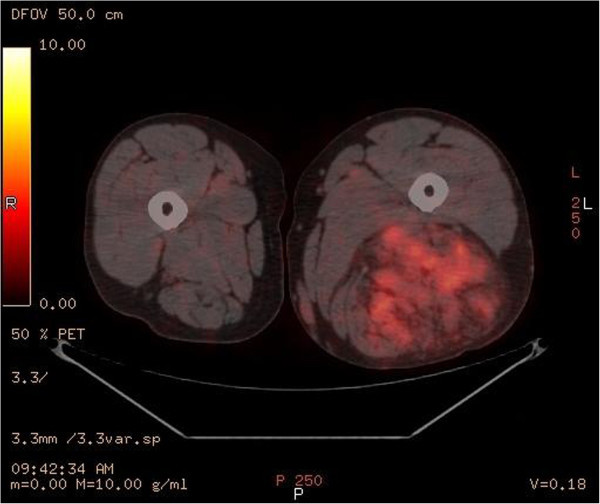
Axial PET-IMG/CT shows hypermetabolic activity with standard uptake of 3.8 to 4.1 in left biceps femoris.
